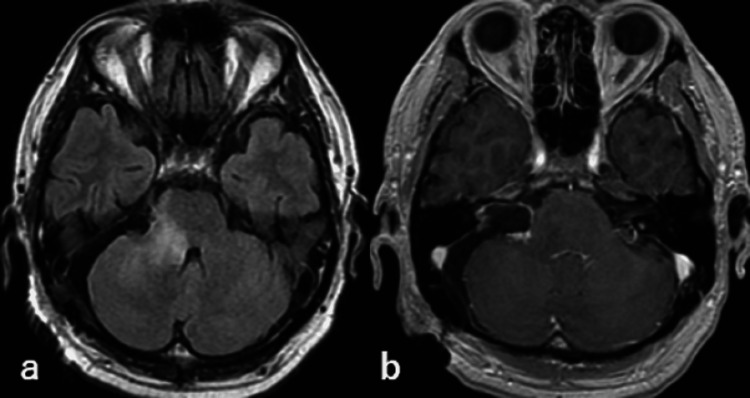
MRI findings on the third postoperative day - (a) FLAIR (b) gadolinium-enhanced MRI: magnetic resonance imaging; FLAIR: fluid-attenuated inversion recovery
